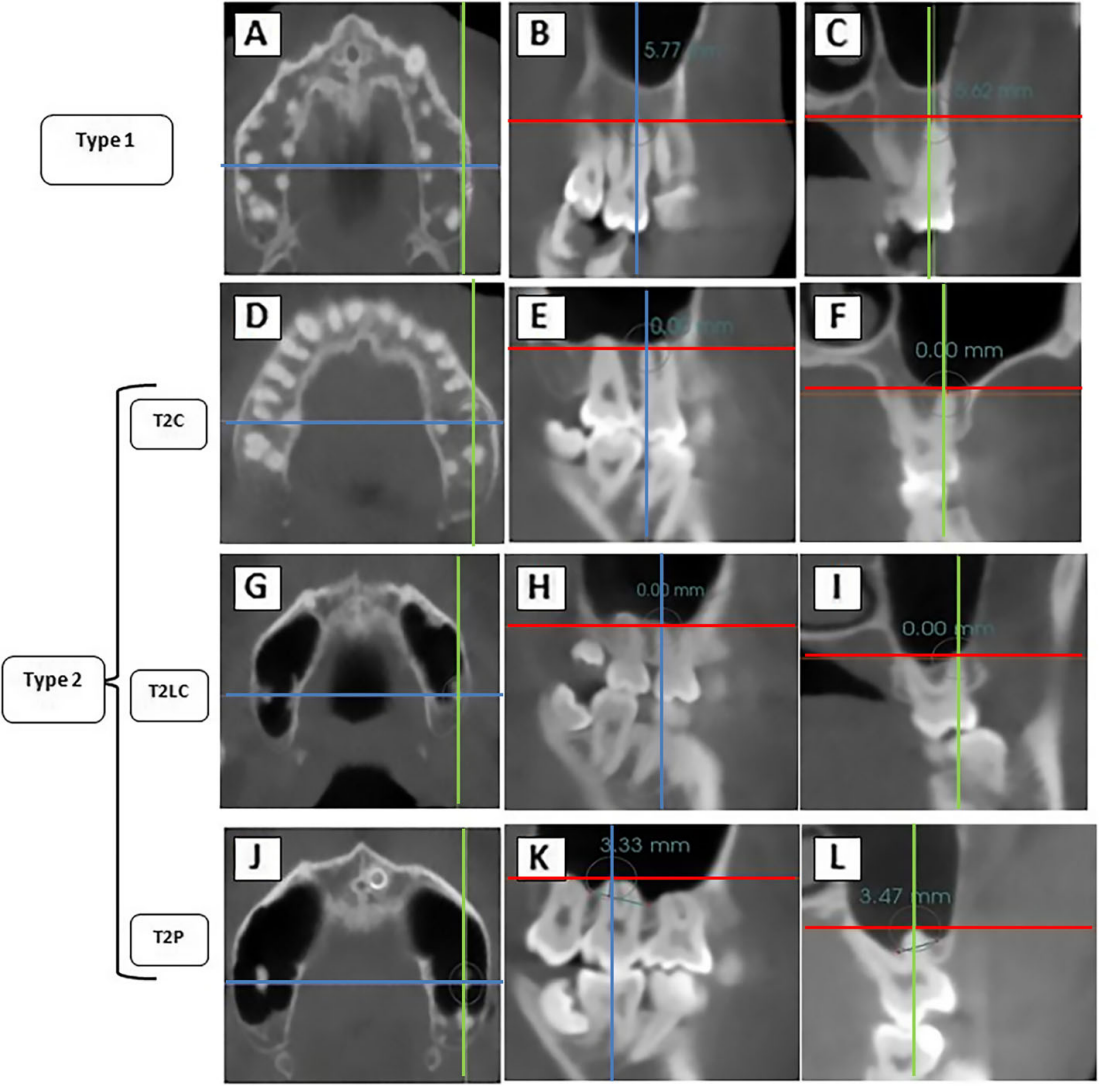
CBCT images demonstrate the three vertical relationships between the maxillary sinus floor (MSF) and the posterior maxillary teeth (PMT). In Axial (A, D, G, and J), sagittal (B, E, H, and K) and coronal planes (C, F, I, and L). Type 1: (A,B and C); Type 2 C (D,E and F). Type 2LC (G, H and I) and Type 2P (J, K, and L)
